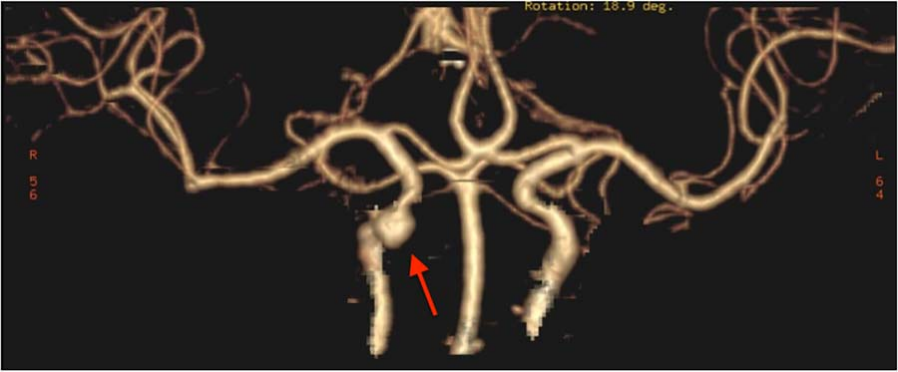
CT angiography, showing pseudoaneurysmal dilatation at the petrous segment of the right ICA.
